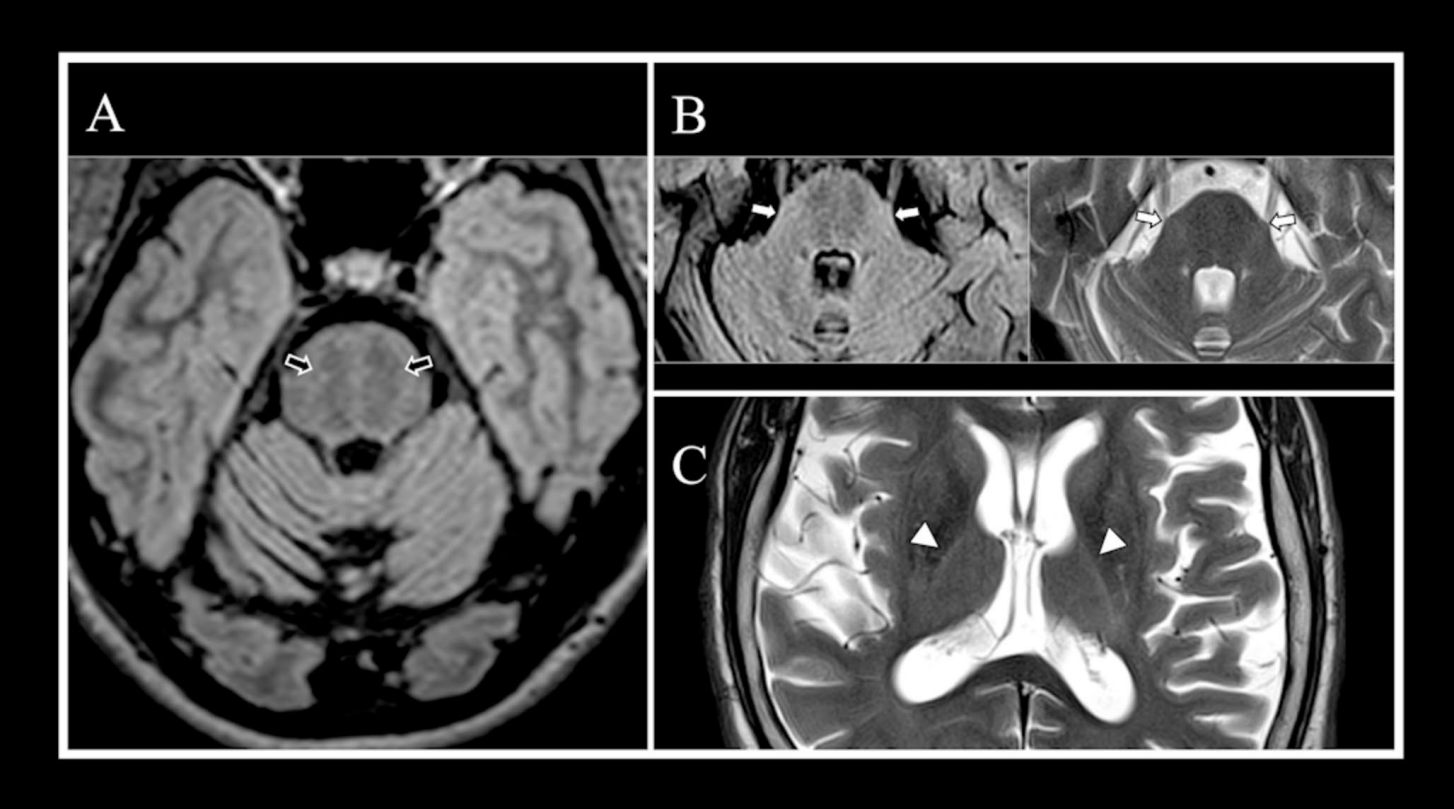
Patterns of signal changes detectable via conventional MRI in ARSACS. Axial multiplanar reconstruction (A) of a 3D Fluid Attenuated Inversion Recovery (FLAIR) sequence showing in an ARSACS patient the occurrence of the “pontine stripes” (*black arrows*). Axial (B) image of 2D FLAIR (*left panel*) showing a mild hyperintensity in the lateral portion of the pons merging in middle cerebellar peduncles, confirmed evaluating the turbo spin echo T2-weighted sequence (*right panel*). On both images, is possible to observe the thickening of middle cerebellar peduncles (*white arrows*). An axial T2-weighted image (C) shows the presence of a bilateral hyperintensity of lateral thalami, described as “thalamic rim sign” (*white arrowheads*)
